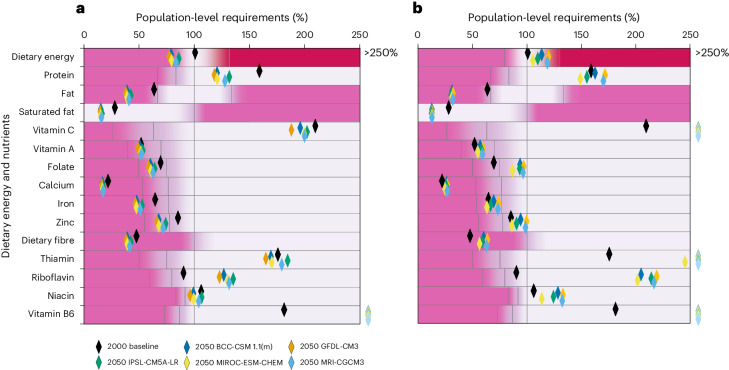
Per capita nutrient supplies with business-as-usual trade, relative to population requirements in Zambia. a,b, Per capita nutrient supplies with business-as-usual trade, relative to population requirements (100%) for HT-RCP2.6 (a) and HT-RCP8.5 (b) in Zambia. Black diamonds indicate baseline (2000) per capita nutrient levels. The five coloured diamonds indicate the projected outcomes in 2050 under different climate models. Grey areas indicate where per capita nutrient requirements are met, and pink areas indicate that requirements are not achieved, with intermediate areas marginal. For all nutrients other than energy and fat, the first threshold represents the Lower Reference Nutrient Intake; the second, the Estimated Average Requirement; the third, the Reference Nutrient Intake (principal target). For fat, thresholds correspond to minimum, min–max midpoint and maximum recommended intakes respectively. For energy, the respective thresholds are minimum, average and maximum dietary energy requirements. The dark pink area indicates where calories are greater than requirements. Vitamin A is measured in retinol activity equivalents. BCC-CSM 1.1(m), Beijing Climate Center, Climate System Model, version 1.1; GFDL-CM3, Geophysical Fluid Dynamics Laboratory Climate Model, version 3; IPSL-CM5A-LR, L’Institut Pierre-Simon Laplace Coupled Model, version 5A, coupled with Nucleus for European Modelling of the Ocean (NEMO), low resolution; MIROC-ESM-CHEM, Model for Interdisciplinary Research on Climate, Earth System Model coupled with atmospheric chemistry; MRI-CGCM3, Meteorological Research Institute Coupled Atmosphere–Ocean General Circulation Model, version 3. Source data
